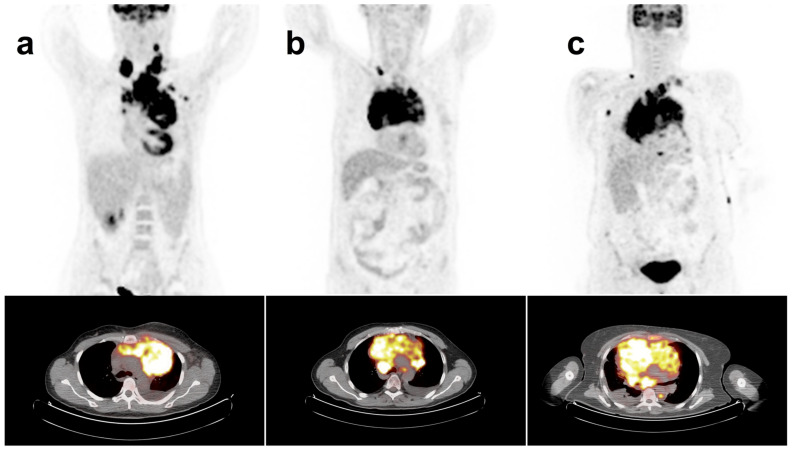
^18^F-FDG-PET/CT maximum intensity projection (MIP) and mediastinal transaxial images show the mediastinal bulky mass in patient with (a) cHL, (b) GZL and (c) PMBCL.
